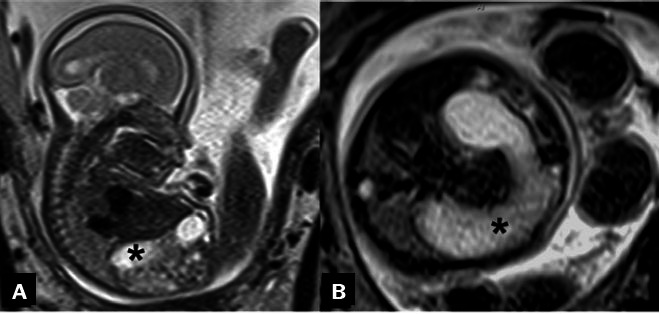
Fetal MRI. Sagittal and axial T2- weighted sequences
